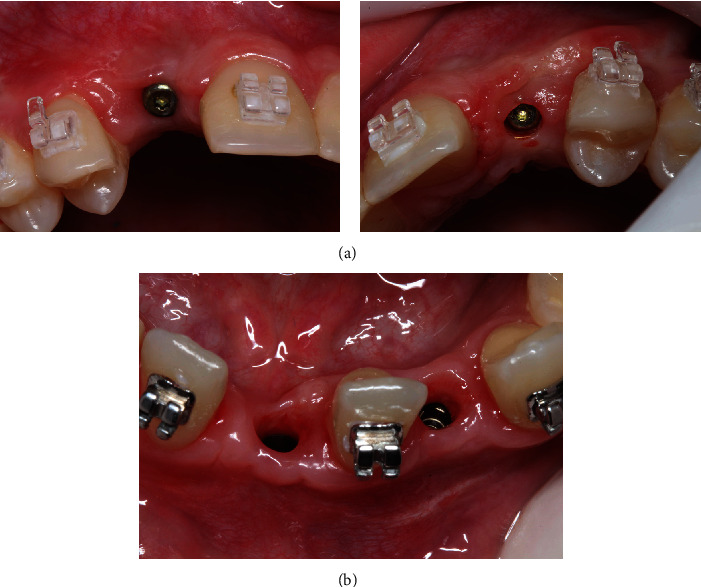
(a) Occlusal view of the lower arch showing the healing process and (b) the periapical radiograph demonstrating the correct placement of the implants.
